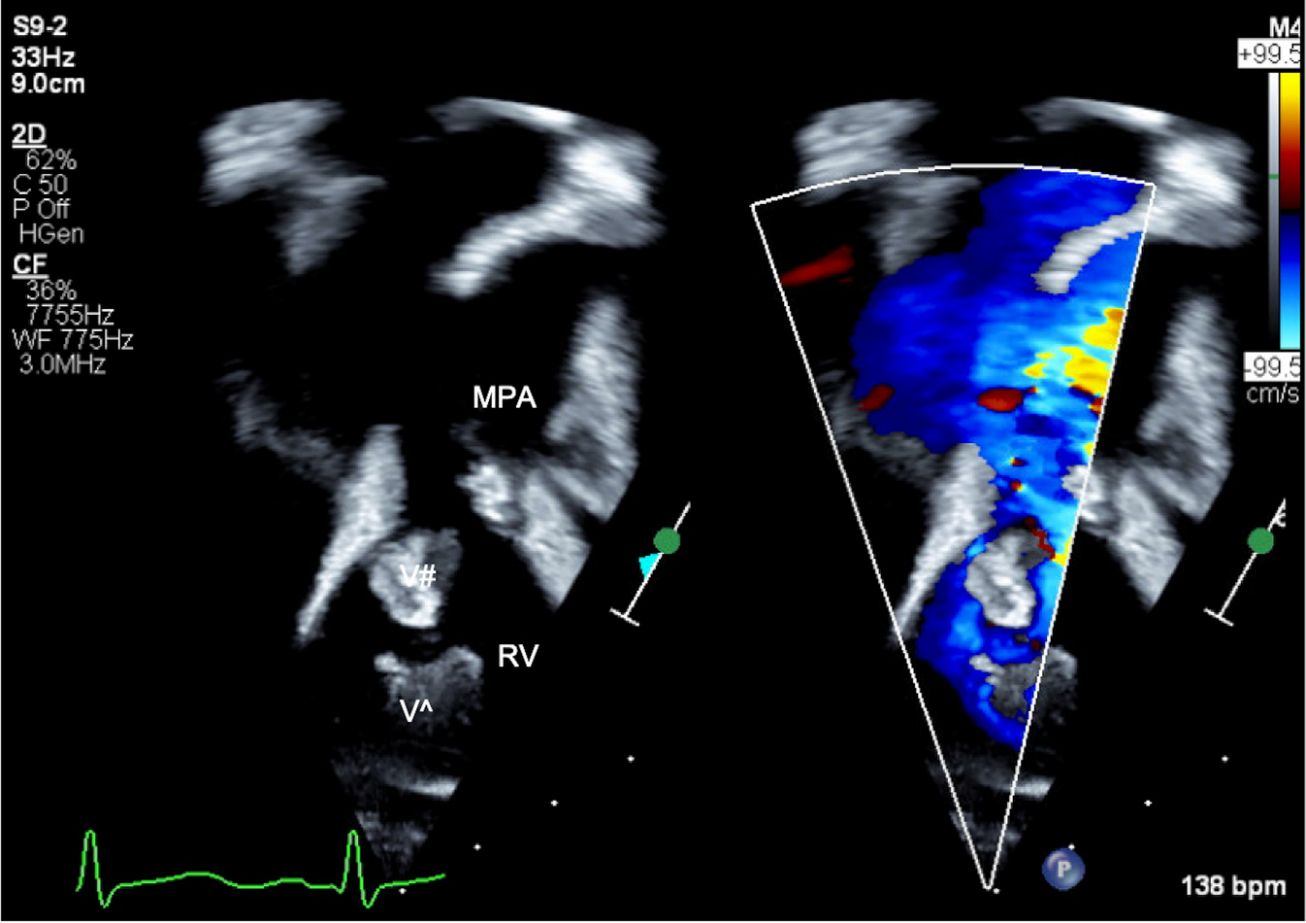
Echocardiogram of Vegetation: Pulmonary Valve Transthoracic apical view focused on the pulmonary valve showing both vegetations on the pulmonary valve (V#) and in the right ventricular outflow tract (Vˆ). MPA = main pulmonary artery; RV = right ventricle.
